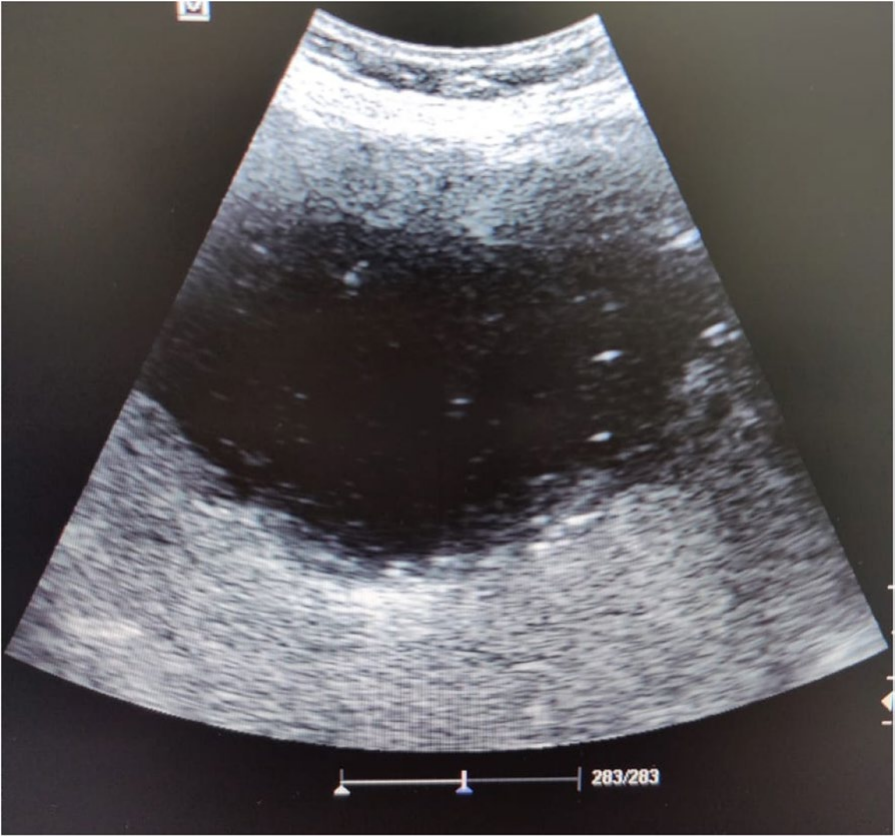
Ultrasonographic picture of the right liver lobe delineating cystic like structure with fine reticulations
